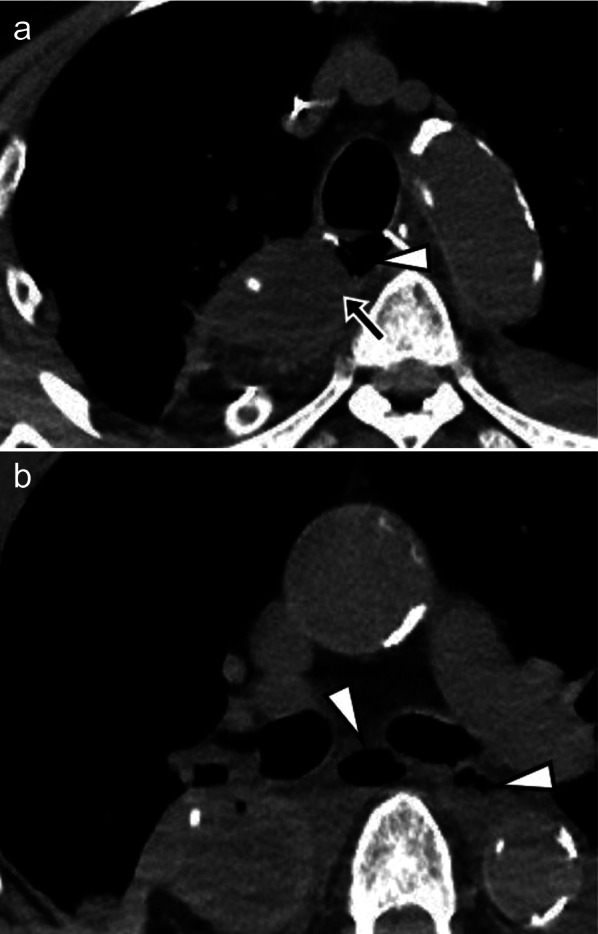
Computed tomography on the 6th postoperative day. a, b Free air (arrowhead) is seen around the anastomotic site (arrow)
